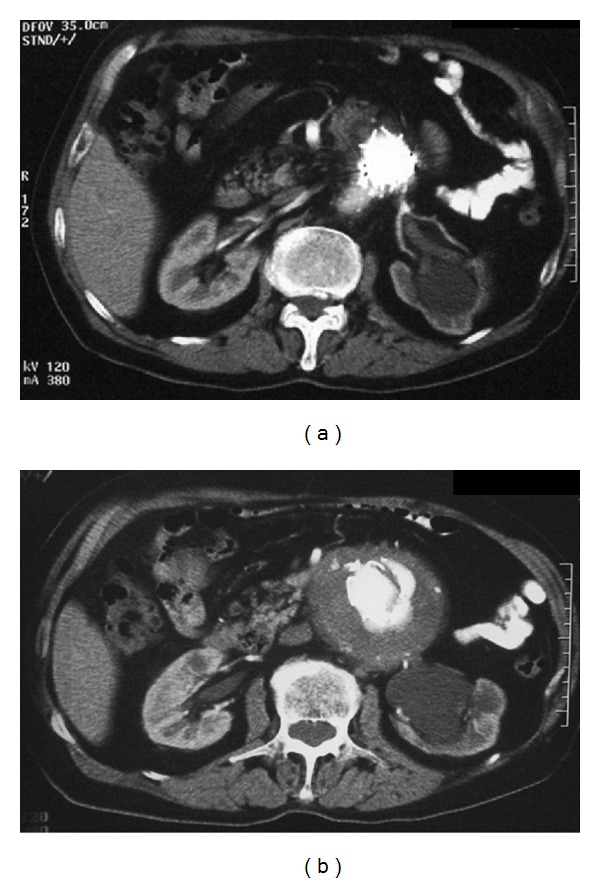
CT scanning one year after EVAR. (a) Migration of the endograft to the straight part of the neck. (b) Endoleak type I detected in the aneurysm.
